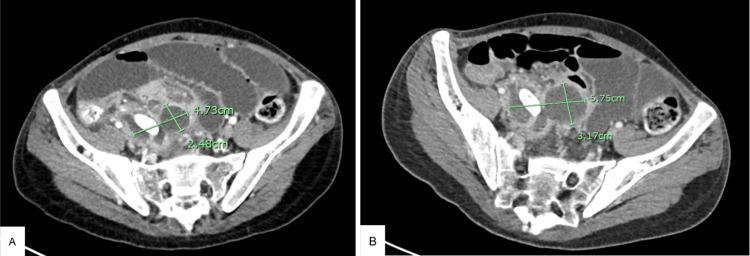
Preoperative CT abdomen and pelvis 2A. Initial imaging revealed perforated appendicitis with a small abscess and small bowel obstruction. (measurements in green) 2B. Repeat imaging revealed perforated appendicitis with a slight interval increase in size of a bilobed periappendiceal abscess. Persistent small bowel obstruction. (measurements in green)
